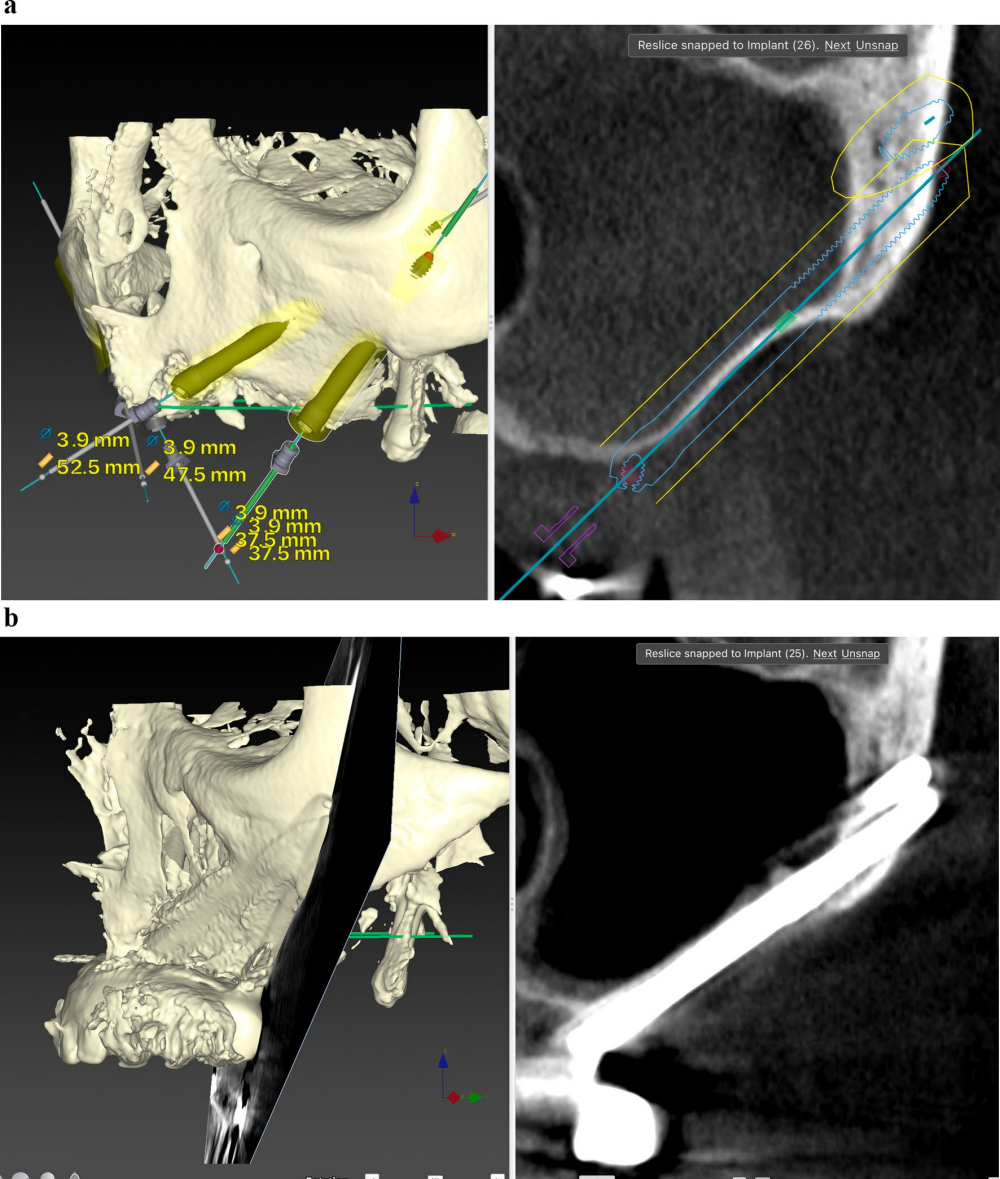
a The oblique CBCT slice shows the left posterior sector with a ZAGA channel osteotomy planning. As in the anterior sector, the maxillary sinus and the ostium are opaque. b The same patient (P6) in a presented this CBCT image showing sinus patency 18 months after a zygoma surgery performed according to the recommendations of the ZAGA protocol and using a Straumann ZAGA Flat implant
